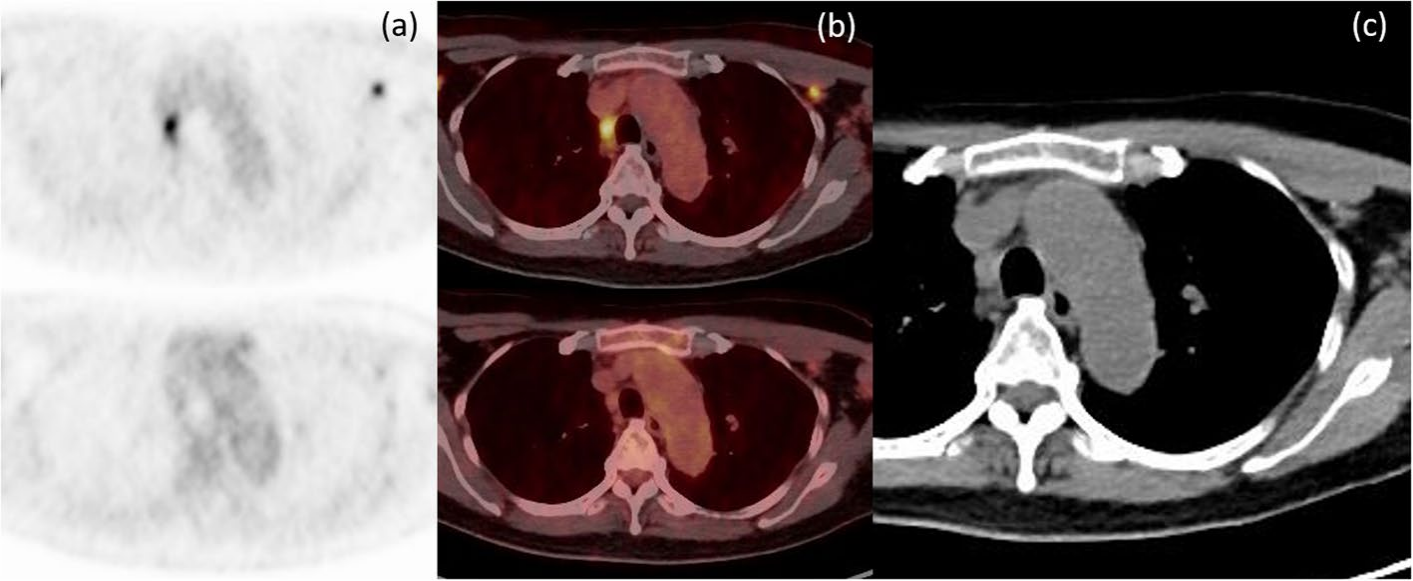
Axial PET a and axial fusion b images of ^18^F-FES (above) and ^18^F-FDG (below) for ^18^F-FES-avid enlarged nodes on CT imaging c without ^18^F-FDG avidity at left axillary and right paratracheal nodes
